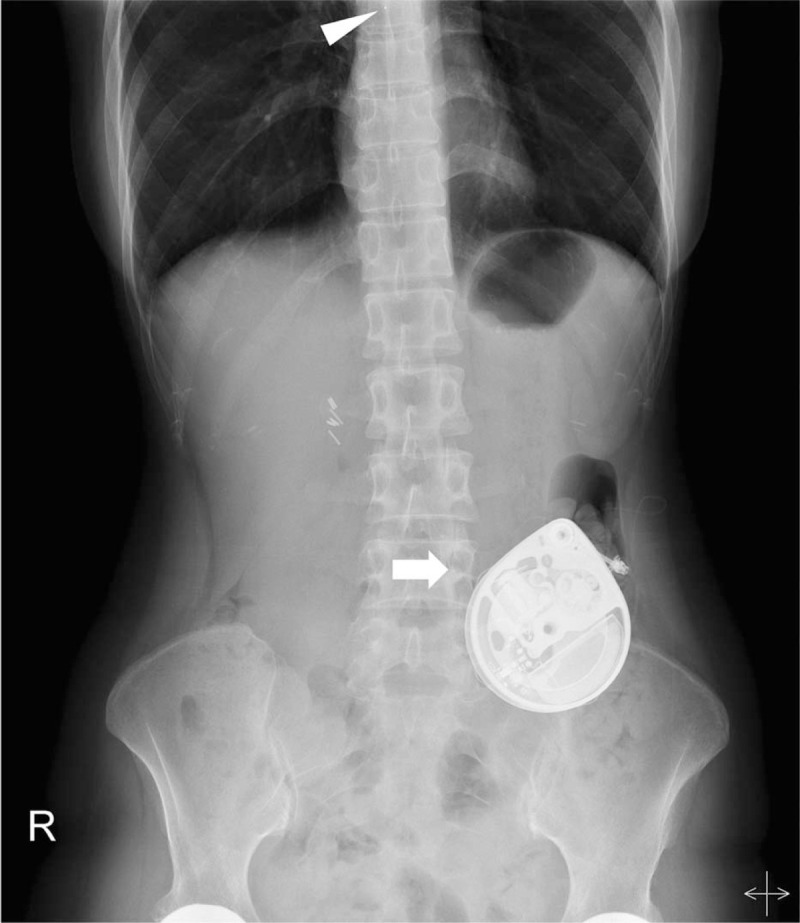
Erect abdominal radiograph taken after the procedure. The arrowhead indicates the catheter tip, and the arrow indicates the pump.
